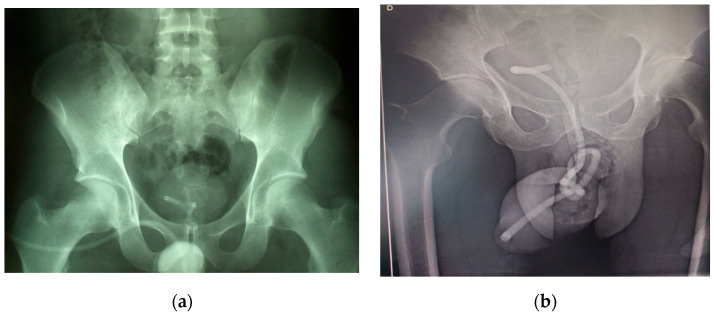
KUB X-ray showing the radiopaque appearance of the intravesical cable: (a) in Case 1, the knot is located at the projection area of the bladder neck, (b) while in Case 2, the knot is positioned more caudally. This is a figure. Schemes follow another format.
